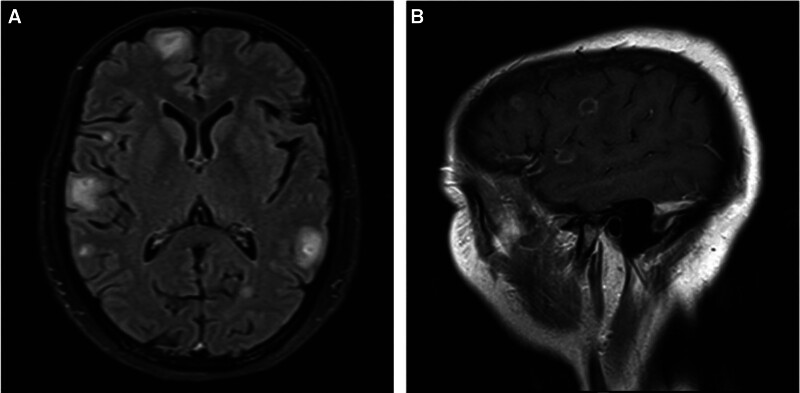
(A) Brain magnetic resonance T2 FLAIR sequence showed multiple lesions in bilateral cerebral hemispheres with high signal, including frontal lobe, temporal lobe, parietal lobe and occipital lobe. (B) T1-enhanced images showed abnormal enhancement of pia mater. T2 FLAIR = T2 fluid-attenuated inversion recovery.
